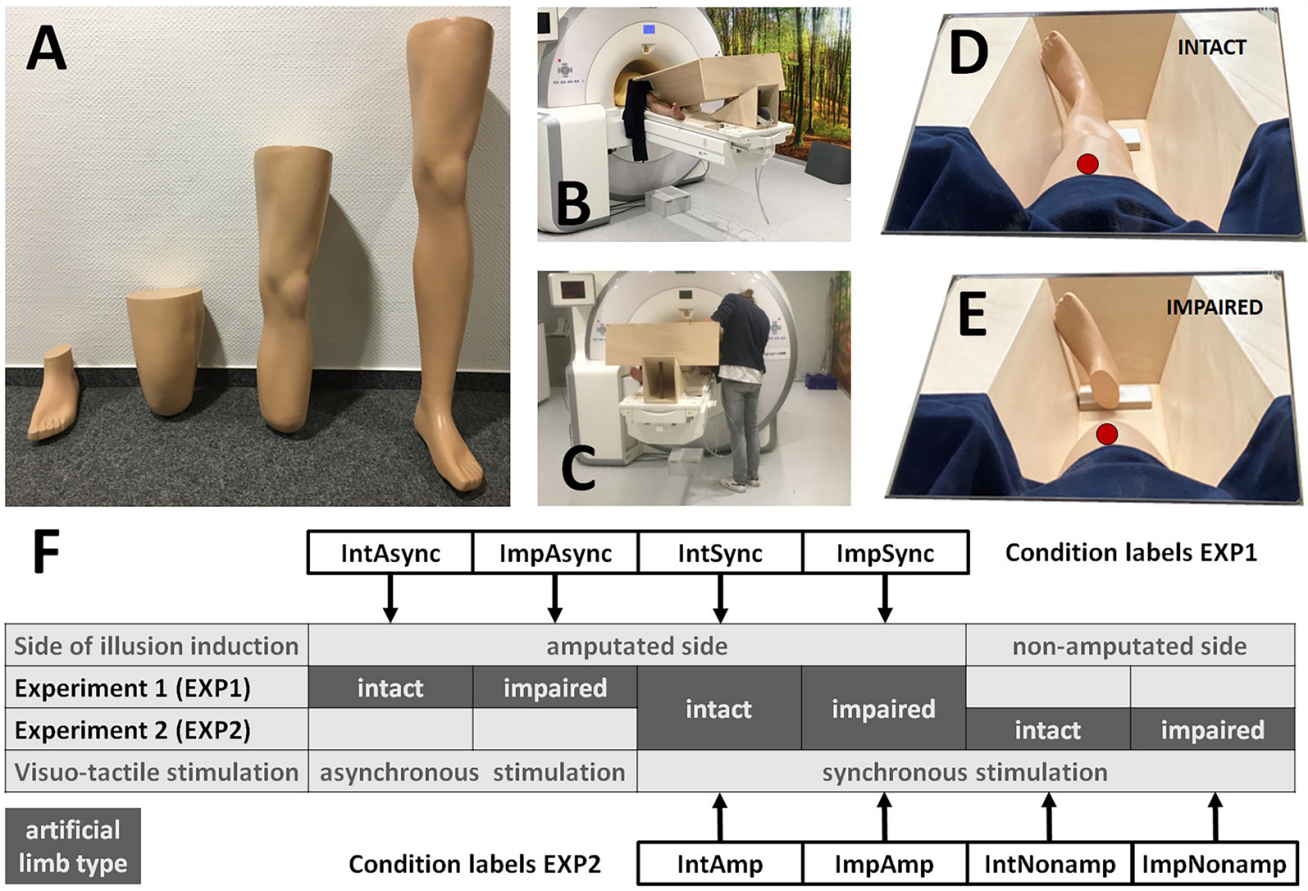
Rubber limb illusion setup. A The set of artificial limbs used in the present study, from left to right: intact limb, residual limb I (transtibial amputation), residual limb II (transfemoral amputation), foot; given are the artificial limbs for the left body side. B Participant’s position in the MRI scanner, with the wooden setup placed between both limbs. C Experimenter’s position during the MRI measurement. D Participant’s perspective during the measurement (intact-condition). E Participant’s perspective during the measurement (impaired-condition). The red markings indicate the area of tactile stimulation and match the respective stimulation area on the participant’s real limb. F Design of the study and allocation of trials to the two experiments. Condition labels are composed of two relevant factors out of the three possible components (side of illusion induction, artificial limb type, visuo-tactile stimulation), depending on which were manipulated in the given experiment. For example, *IntAsync* refers to an intact (= *Int*) artificial limb under asynchronous (= *Async*) visuo-tactile stimulation; since Experiment 1 was conducted only on the amputated side, side of illusion induction is not further specified in the condition label. And *ImpNonamp* refers to an impaired (= *Imp*) artificial limb with the illusion induced on the non-amputated (= *Nonamp*) side; since Experiment 2 only applied synchronous touches, visuo-tactile stimulation is not further specified in the condition label
